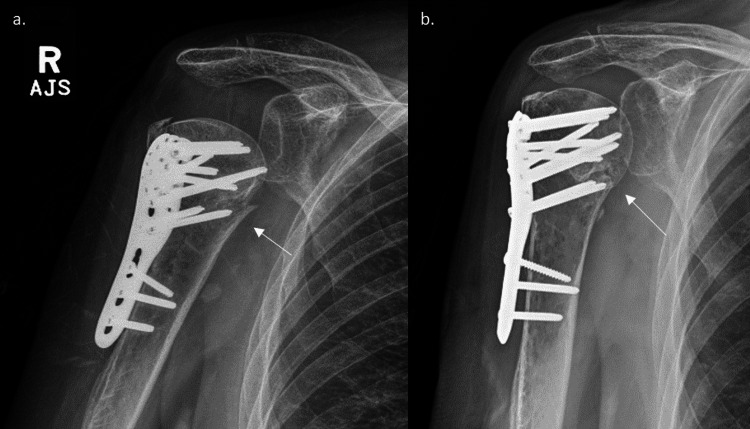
Postoperative anteroposterior radiographs at (a) one month postoperative and (b) seven months postoperative showing solid union of the fracture The white arrows show the approximate position of the arterial repair.
